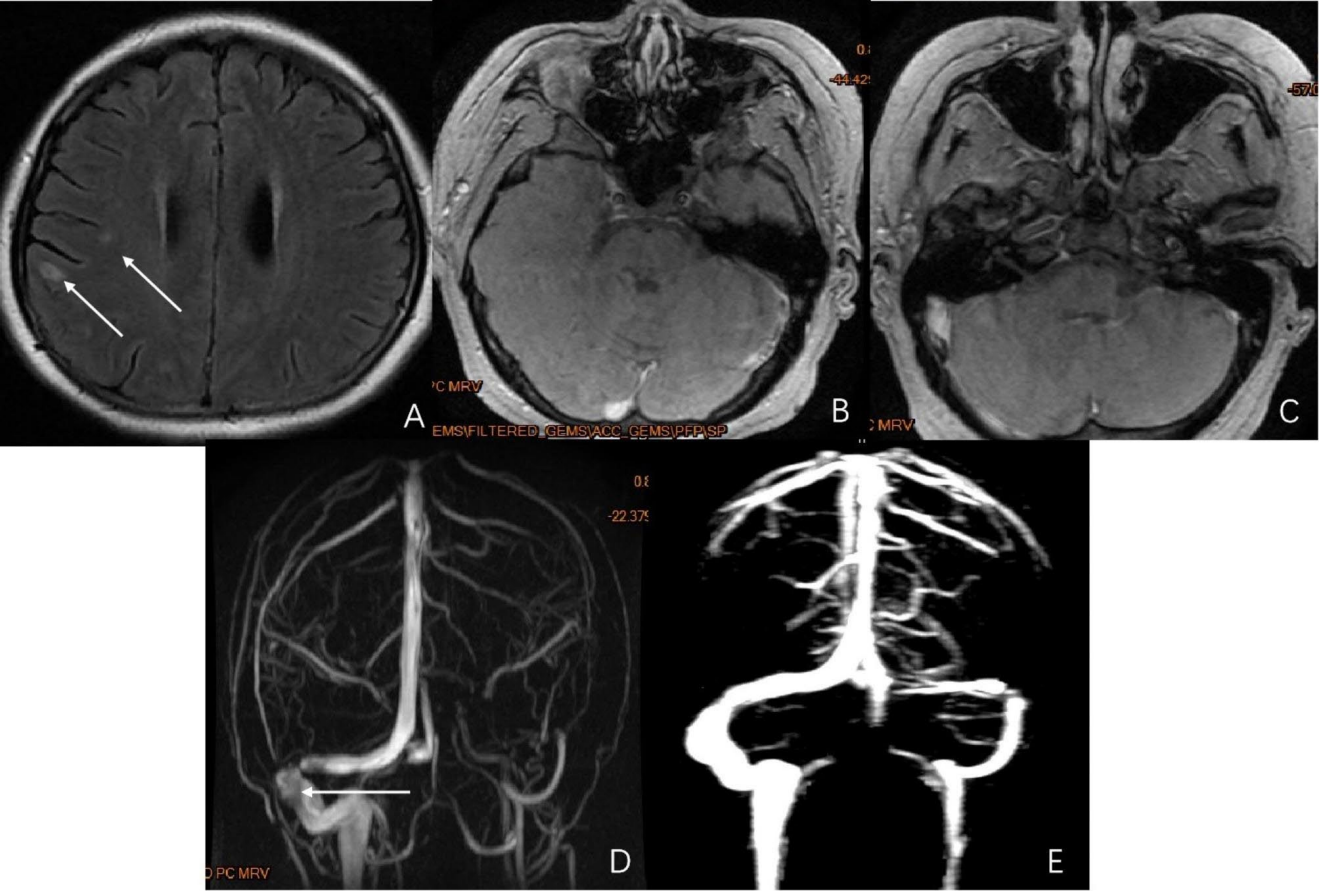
Radiographic findings of the patient in case 2. A: Brain plain MRI FLAIR sequence shows multiple high-signal intensity patches in bilateral frontal parietal cortex and subcortical white matter. B: MRV shows a locally narrowed right sigmoid sinus, while the left transverse sinus and sigmoid sinus are not shown. C and D: Post-contrast MRI T1WI shows filling defects in the right sigmoid sinus, left transverse sinus, and sigmoid sinus. E: After 6 months, the re-examination image shows that the right sigmoid sinus is normal, and the filling defect of the left transverse sinus and sigmoid sinus was better than before
